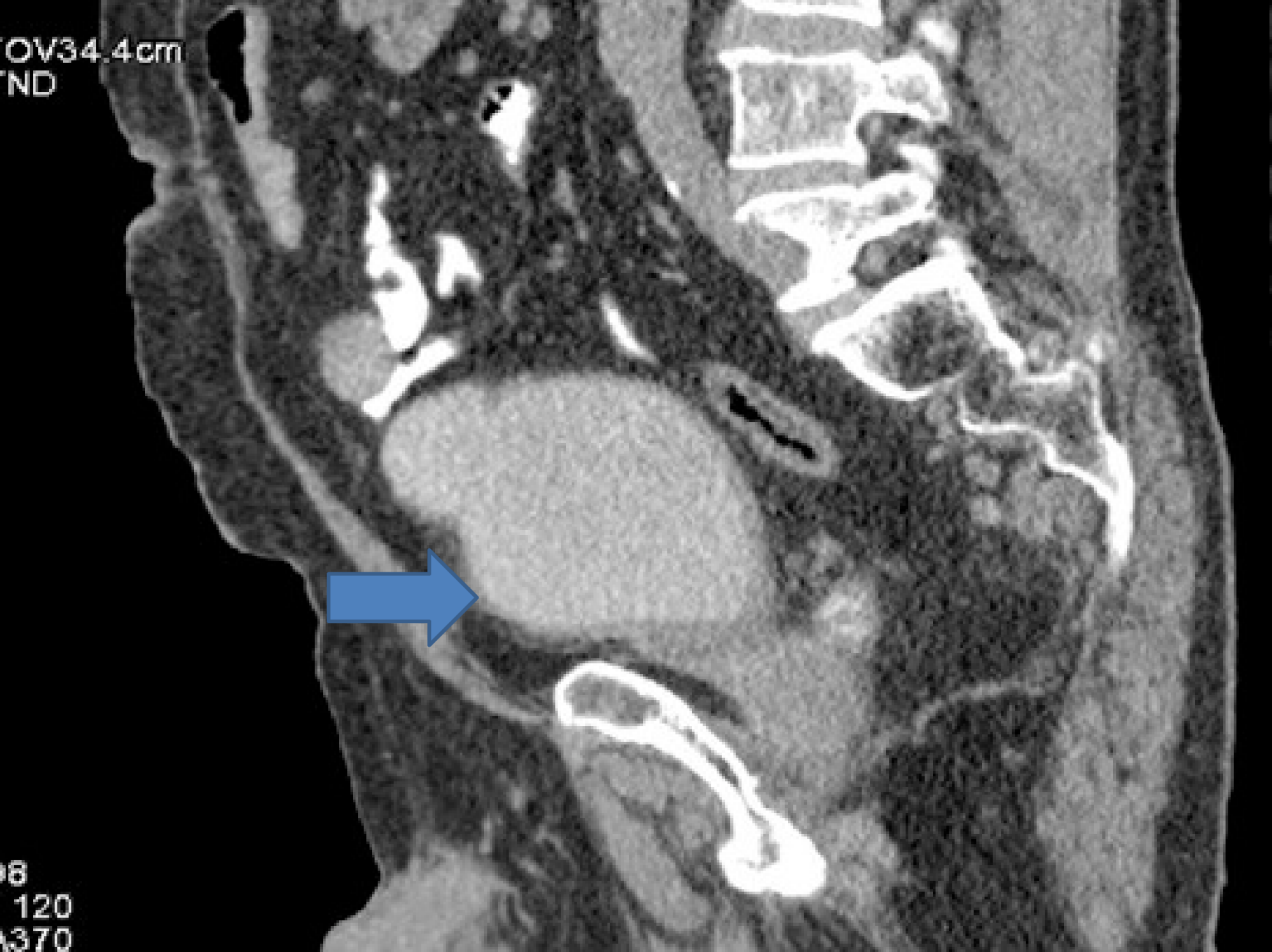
Computed tomography (CT) done after 17 months of starting radiation and immunotherapy showing complete remission in urinary bladder.
